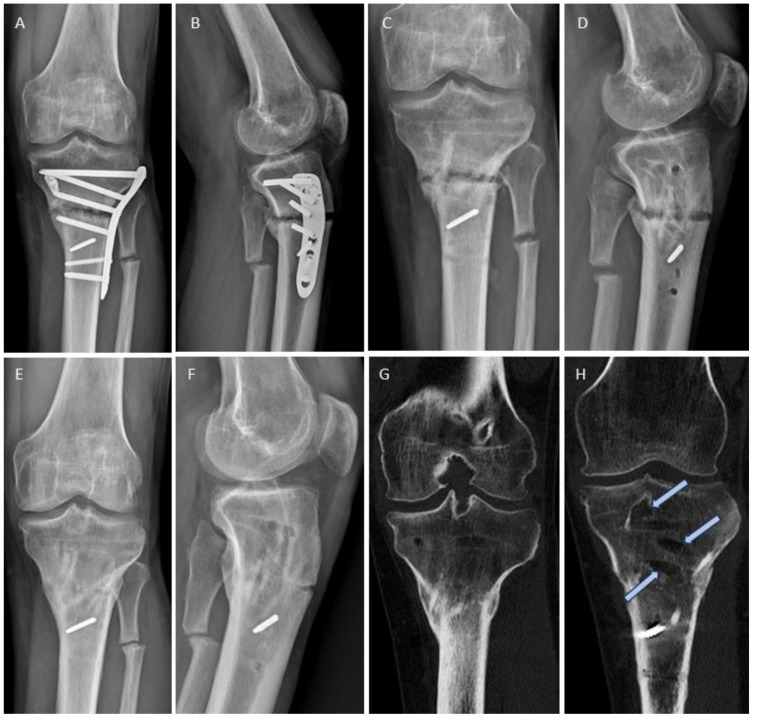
Non-union following a high tibial osteotomy in a 41-year-old smoker in October 2021. (A,B) Despite extracorporeal shock wave therapy and parathyroid hormone therapy, union was not obtained. The patient experienced severe pain persisting day and night, with limited weight-bearing capacity for short distances. There was a knee extension deficit of 25°. The osteotomy was clinically unstable, opened laterally and medially by approx. 10°, and there was a peroneal lesion with numbness of the lower leg laterally. The patient insisted on metal removal and categorically opposed any future metal treatment. (C,D) In July 2022, revision surgery with hardware removal, non-union debridement, and axis correction including reclination was performed. The non-union was bridged with an allogeneic bone block and fixed with six Shark Screws^®^. The large bone defects were filled with allogeneic bone paste (DBM putty). (E,F) Good consolidation of the tibia 14 months after revision. The new bone formation was supported by the bone structure of the allogeneic bone screw, which facilitates cell migration and revascularization. The Shark Screws^®^ have become nearly imperceptible. Bone healing was obtained after 16 weeks. (G,H) CT scans 15 months after revision. The Shark Screws^®^ have undergone complete remodeling, making them indistinguishable from the host bone (G). However, the bone voids resulting from the removal of metal hardware ((H), clear blue arrows) have not yet been filled with new bone, even after 15 months.
